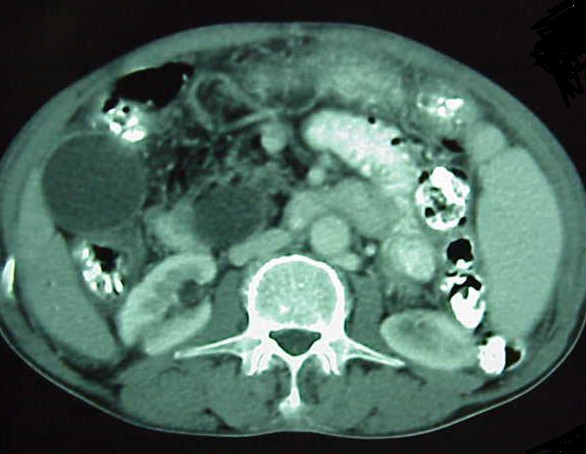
CT scan before treatment. Dilatation of extra- and intrahepatic bile ducts and heterogeneity of the head of the pancreas are visible.
